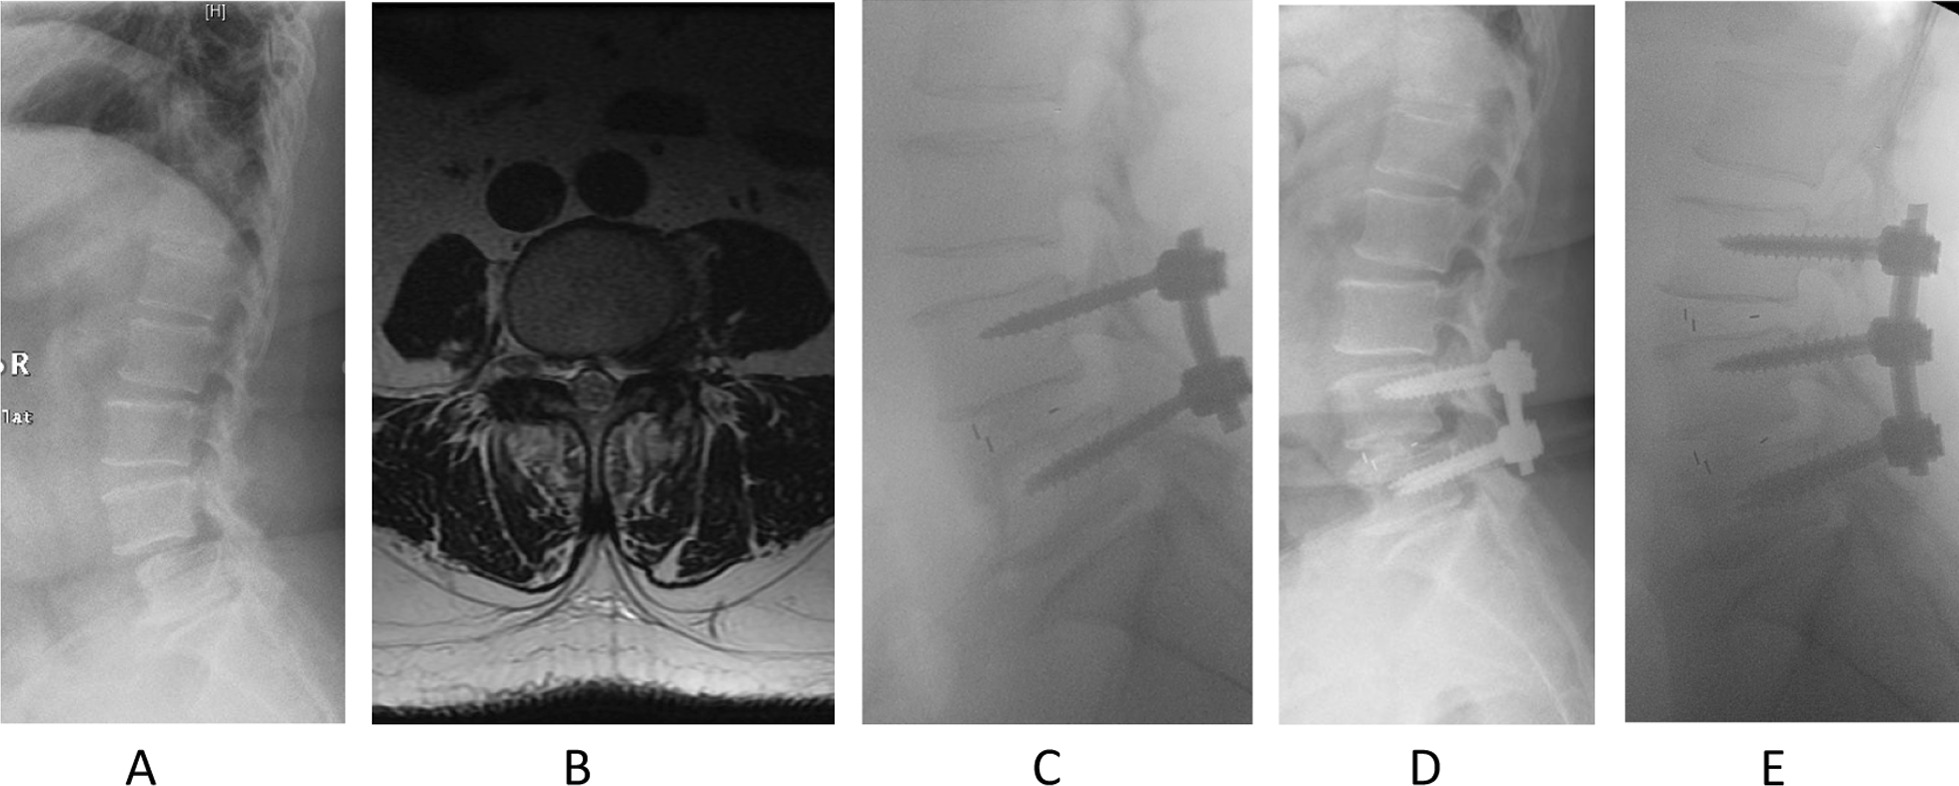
A 63-year-old female underwent L4–5 transforaminal lumbar interbody fusion surgery in 2016 for lumbar spondylolisthesis and lumbar spinal stenosis and underwent L3–4 revision surgery for adjacent segment disease 4 years later. A shows the lumbar lateral plain radiograph before the first surgery. B suggests significant fatty infiltration of the multifidus muscle at the L4–5 segment before the first surgery. C shows the lumbar lateral plain radiograph after the first surgery. D and E are pre- and postoperative radiographs of the revision surgery, respectively
